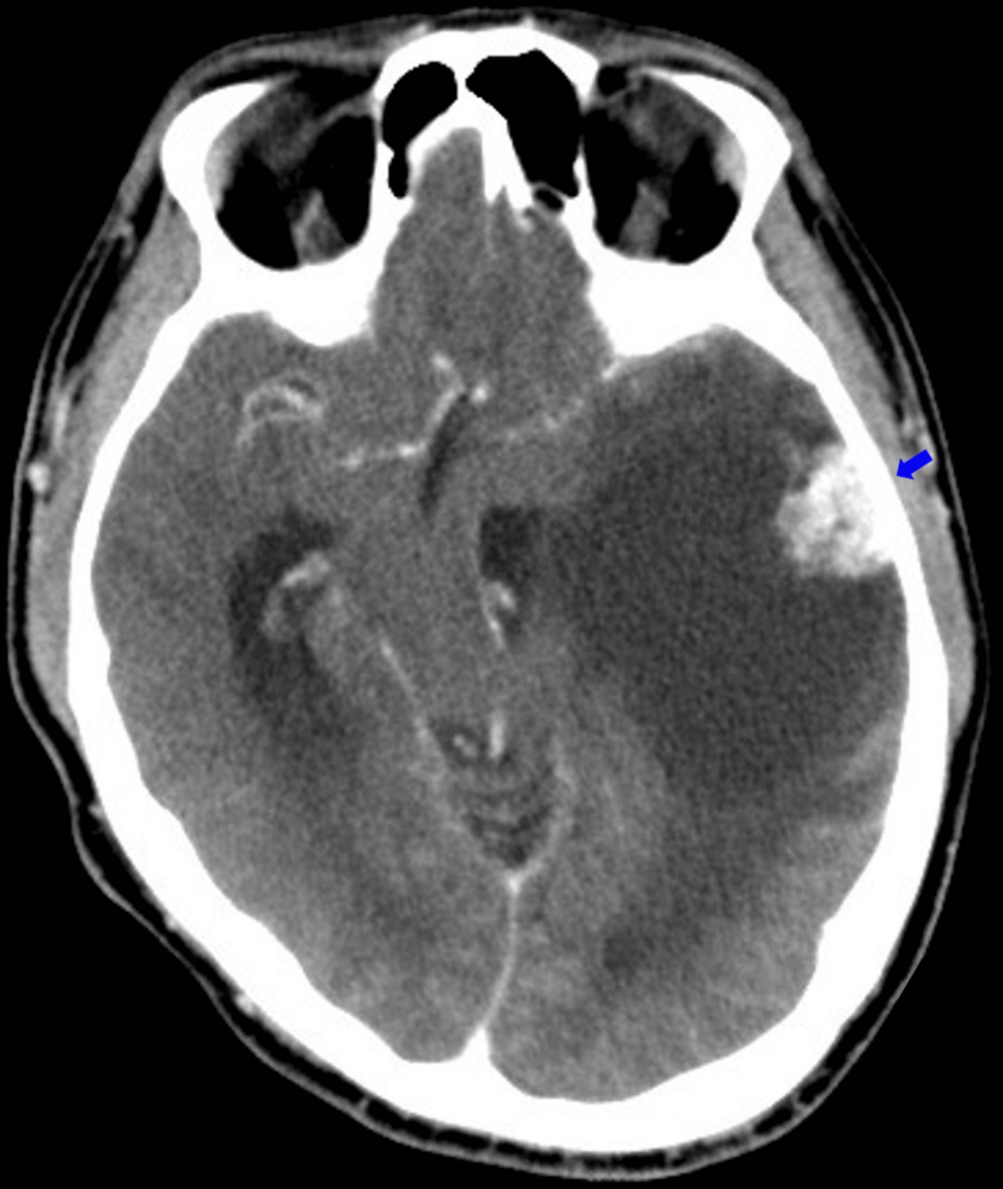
Brain CT with contrast of case 1 A tumor was identified in the left temporal lobe, with a peripherally located solid component showing contrast enhancement (blue arrow). The cystic component caused significant mass effect, resulting in midline shift to right side and uncal herniation.
